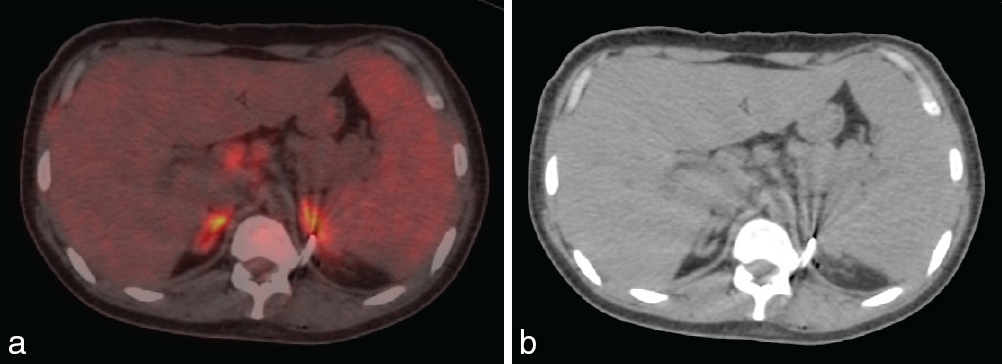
Fused transaxial positron emission tomography/CT (a) and CT (b) images showing the tip of the percutaneous biopsy needle precisely positioned in the left adrenal gland under robotic arm guidance.
